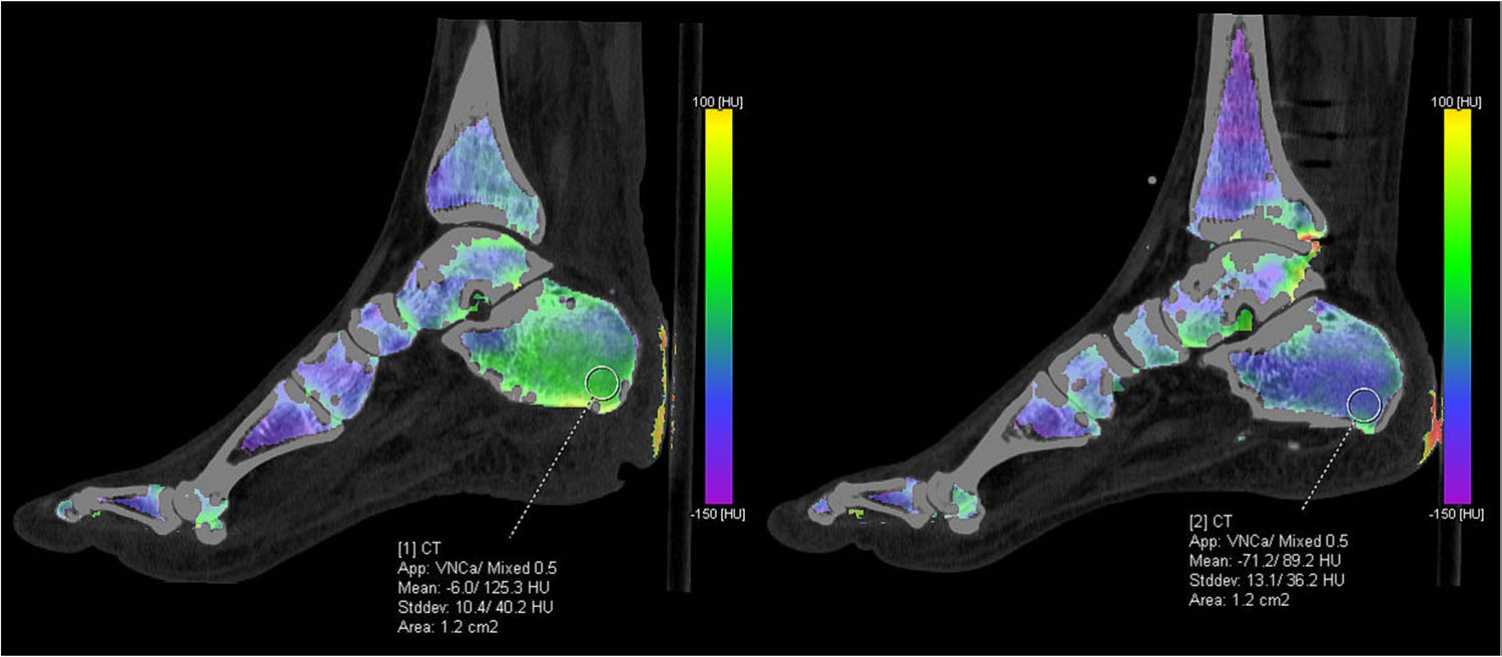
Measurement of CT values in the calcaneus on the ulcer side (left) and the reference side (right)
